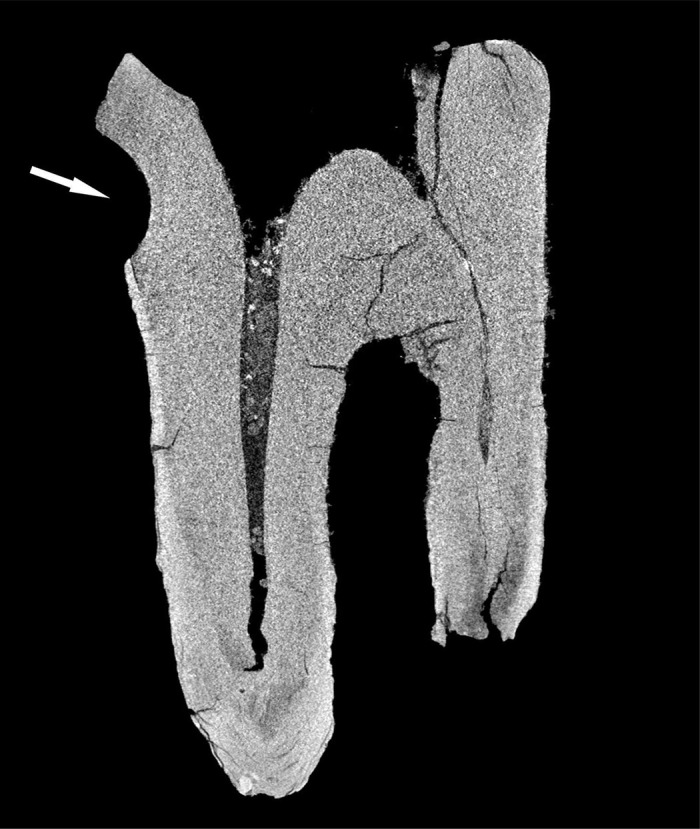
Chagyrskaya 64, CT-scan. Arrow indicates a toothpick groove.
